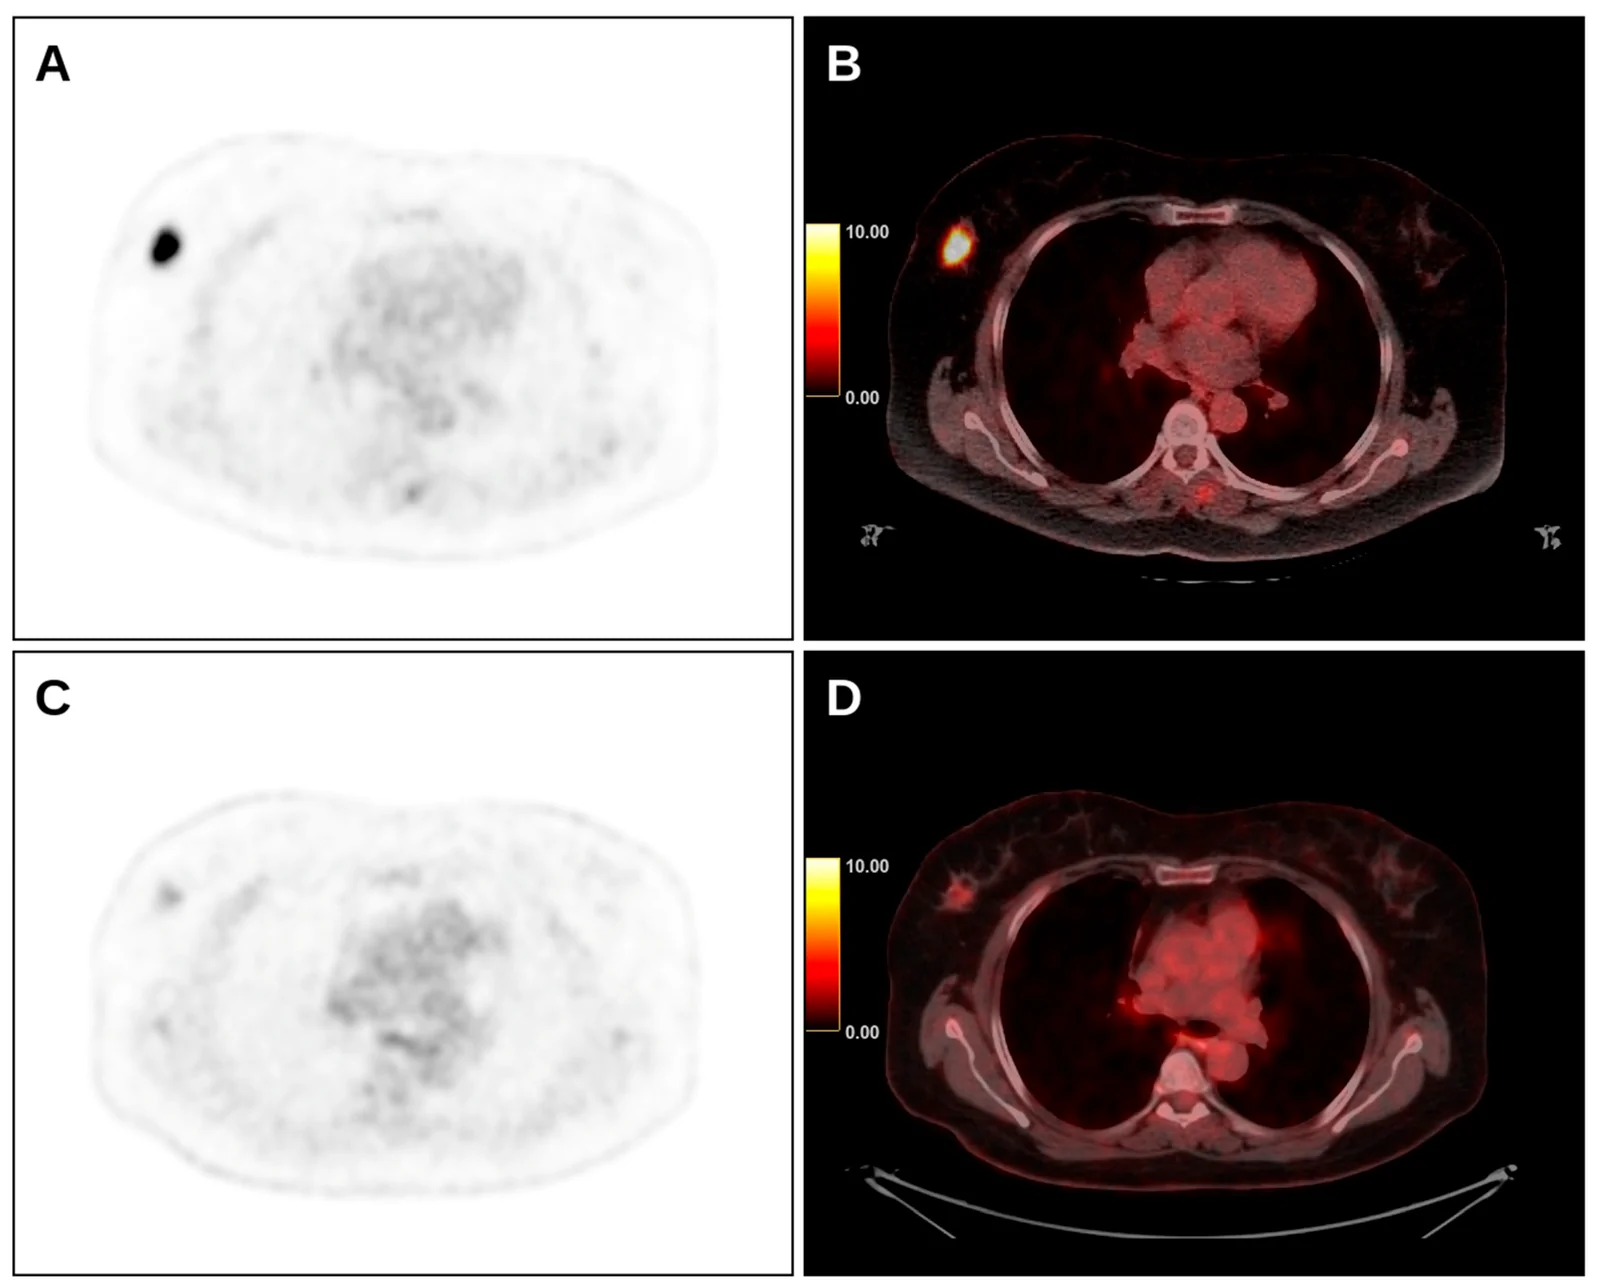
Representative PET/CT images of a patient who achieved a pCR following NST. Baseline PET (A) and fused PET/CT (B) images demonstrate an irregular right breast mass with intense FDG uptake. Post-treatment PET (C) and fused PET/CT (D) images show mild residual focal FDG uptake within the tumor bed, which was interpreted as residual metabolic activity. However, pathological examination revealed a complete pathological response despite the presence of mild residual FDG uptake. The calculated ΔSUVmax was 82%.
